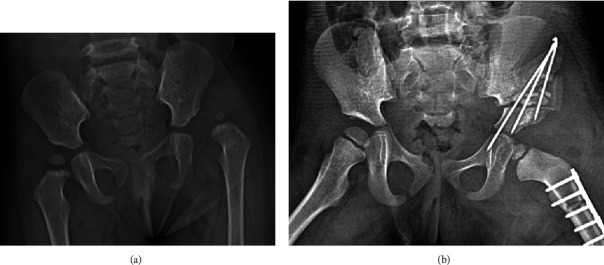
Preoperative and postoperative imaging data of a 25-month-old girl with DDH. (a) Anteroposterior radiograph of the pelvic before surgery. (b) Anteroposterior radiograph of the pelvic after surgery.
